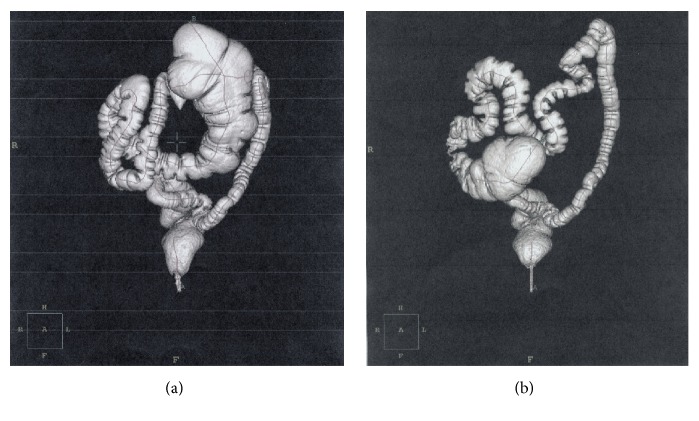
(a) Virtual colonoscopy. Three-dimensional volume-rendered CT colonography (anteroposterior view) showed an elongated right colon with the cecum dislocated in the upper left quadrant. (b) Virtual colonoscopy one year after surgery. Three-dimensional volume-rendered CT colonography (anteroposterior view) demonstrated the cecum and the ascending colon in the physiological position.
